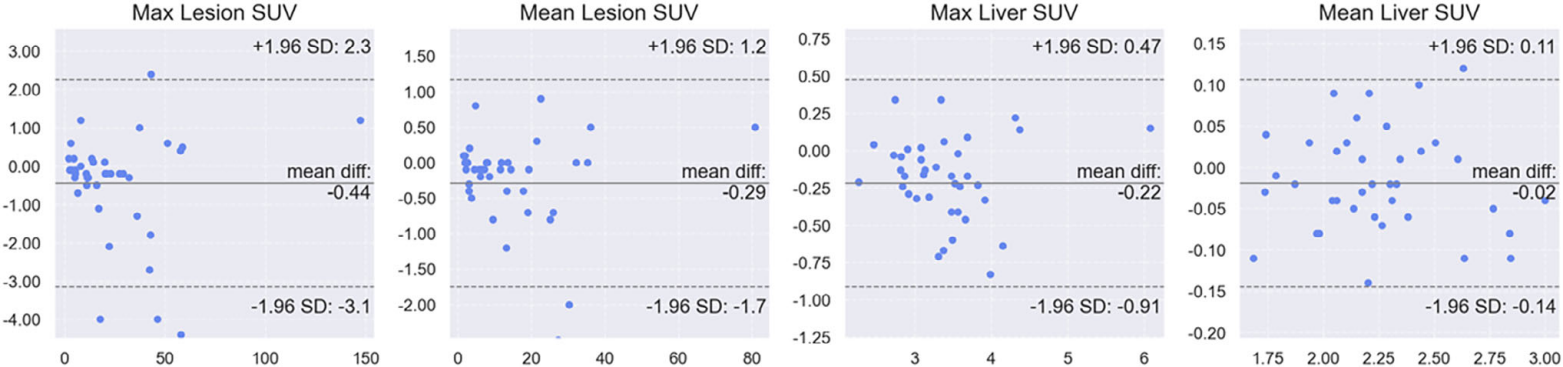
Bland‐Altman analysis of SUV for lesion and liver. Where solid line in the middle and two dashed lines aside are mean difference and ± 1.96 standard deviations, region with two dashed line is with 95% limits of agreement (LOA). x and y axis are mean value and difference value each.
